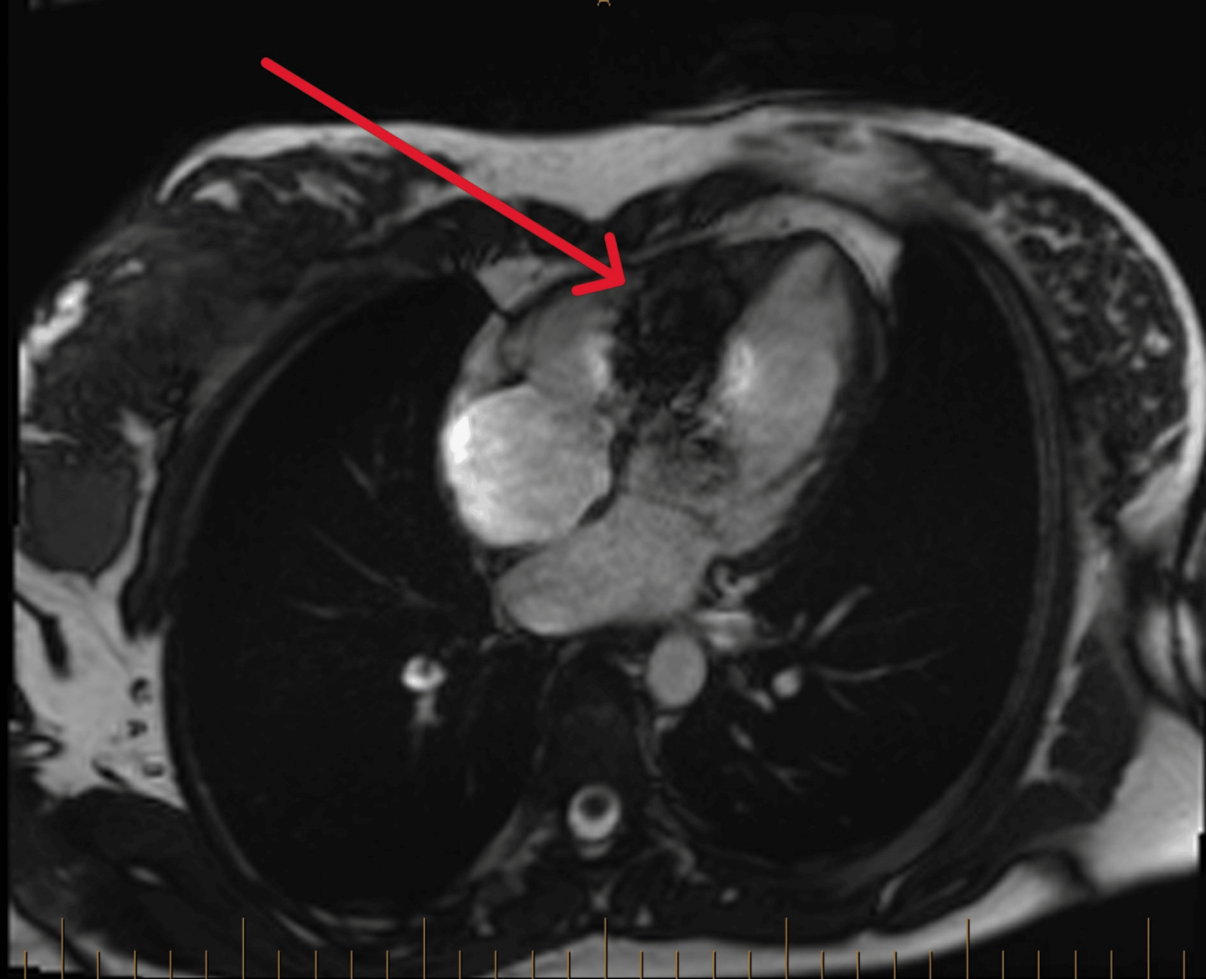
Cardiac MRI with the red arrow pointing towards the area of artefact created from the leadless pacemaker.
